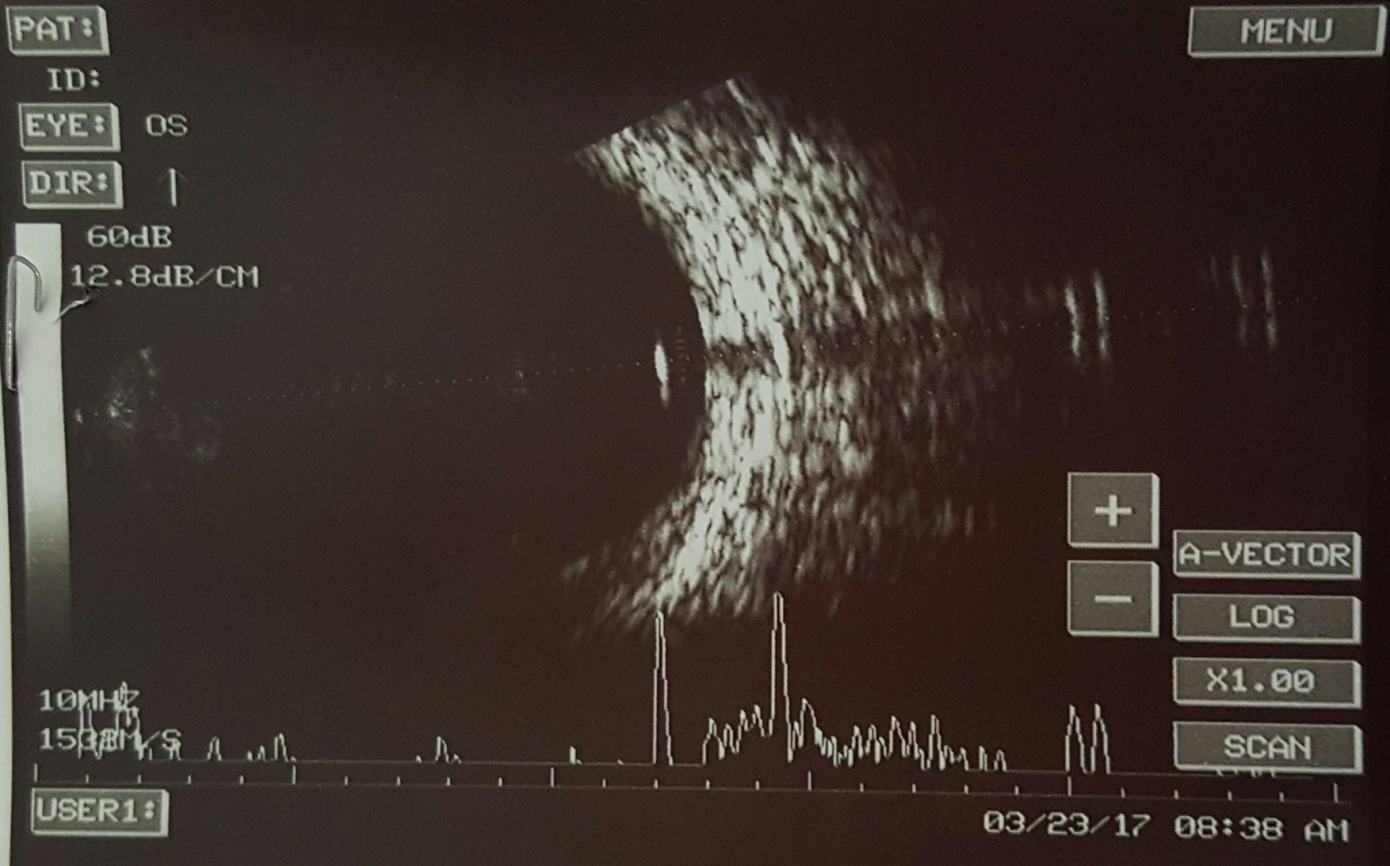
Ultrasonography following the initial surgery showing a hyperintense lesion with backshadowing suggestive of a foreign body
